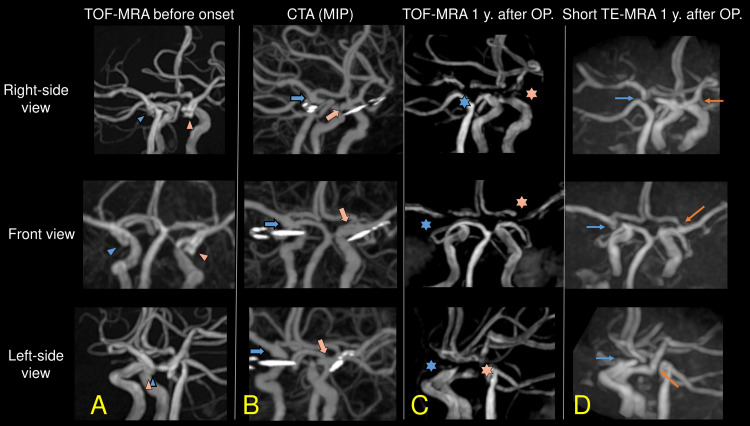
Comparison of each MRA sequence TOF-MRA before the subarachnoid hemorrhage onset revealed the 7 mm aneurysm at the left ICA (red arrowheads, A) and the 5 mm aneurysm at the right ICA (blue arrowheads; A). On the third postoperative day after clipping, CTA revealed the clips and bilateral ICAs (thick red and blue arrows; B). About a year after the clipping, 1.5T TOF-MRA did not describe the bilateral ICAs, nor some parts of MCAs and ACAs (red and blue asterisks; C). Short TE-MRA did not describe the right ICA (thin blue arrows, D), but it revealed the right MCA and A1 portion of the ACA. Also, it revealed those on the left (thin red arrows, D). Abbreviations in the figures: CTA; computed tomography angiography, OP; operation, MIP; maximum intensity projection, TE; echo time TOF-MRA; time-of-flight magnetic resonance angiography
